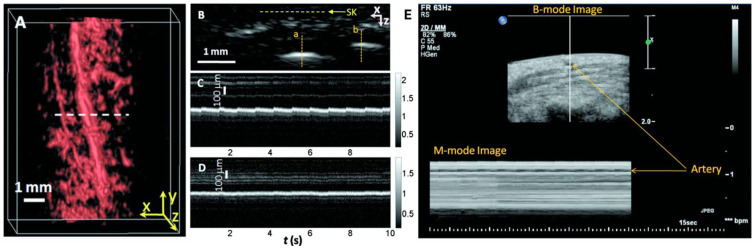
Dynamic in vivo photoacoustic and ultrasound imaging using a PACT system (reproduced with permission from Ref. [33]). (A) Volumetric photoacoustic image of a human hand, with dashed line indicating the cross-section for real-time B-scan imaging. (B) B-scan image corresponding to the horizontal dashed line in (A), showing skin surface (SK). (C) M-mode image along vertical line a in (B), demonstrating time-resolved arterial pulsation. (D) M-mode image along vertical line b in (B), showing venous dynamics. (E) Corresponding ultrasound images of arterial pulsation.
